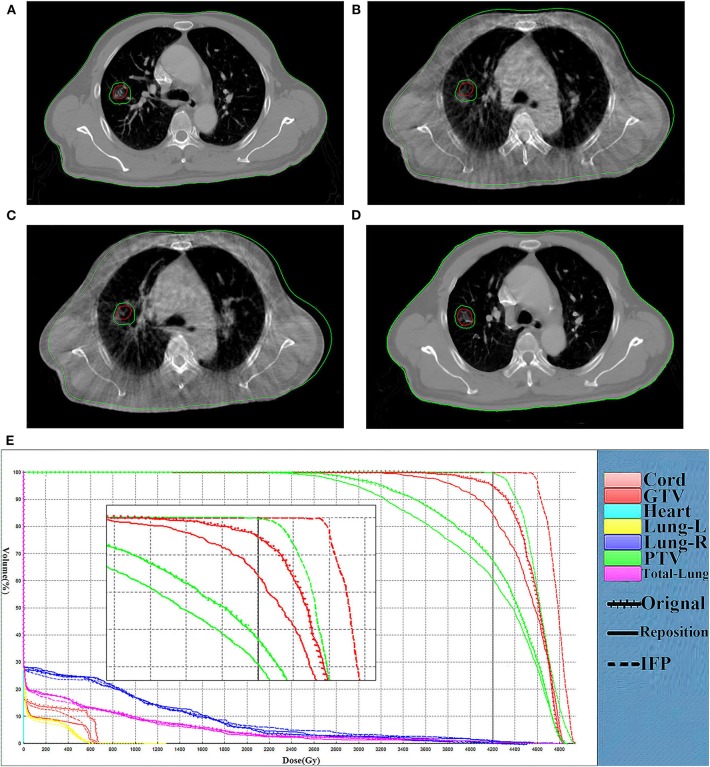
Comparison of representative CT slices. Comparison of representative CT slices obtained on the planning CT (A), the CBCT before shift couch (B), the CBCT after shift couch (C), and the corrected CT image (D) as well as DVH curves comparison (E) of the same fraction for a lung cancer patient. The dotted, dashes, and solid curves represent the original, IFP and the repositioning plans, respectively.
